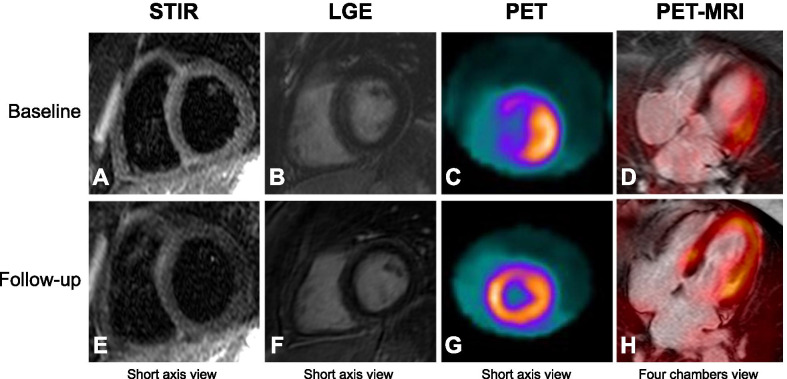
50-year-old female (patient #7) with negative STIR (A), no LGE (B) and increased ^18^F-FDG uptake in the lateral region of the LV (C) and corresponding fused images (D) at baseline study. Unremarkable PET-MRI findings at follow-up study (E–H)
